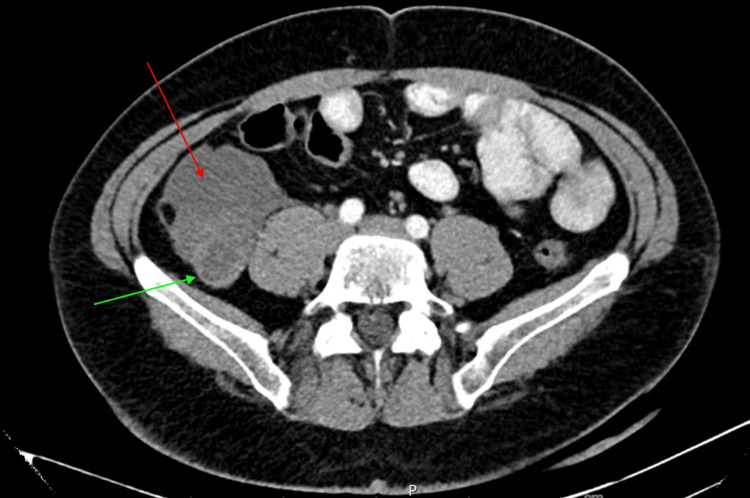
CT abdomen and pelvis with IV and oral contrast, axial image, demonstrates a significantly dilated appendix (marked by a green arrow), measuring up to 2.4 cm in diameter, indicative of an abnormal distension. Adjacent to the appendix, a complex fluid collection (highlighted by the red arrow) is noted, raising concern for a ruptured appendicitis or a ruptured appendiceal mucocele.
